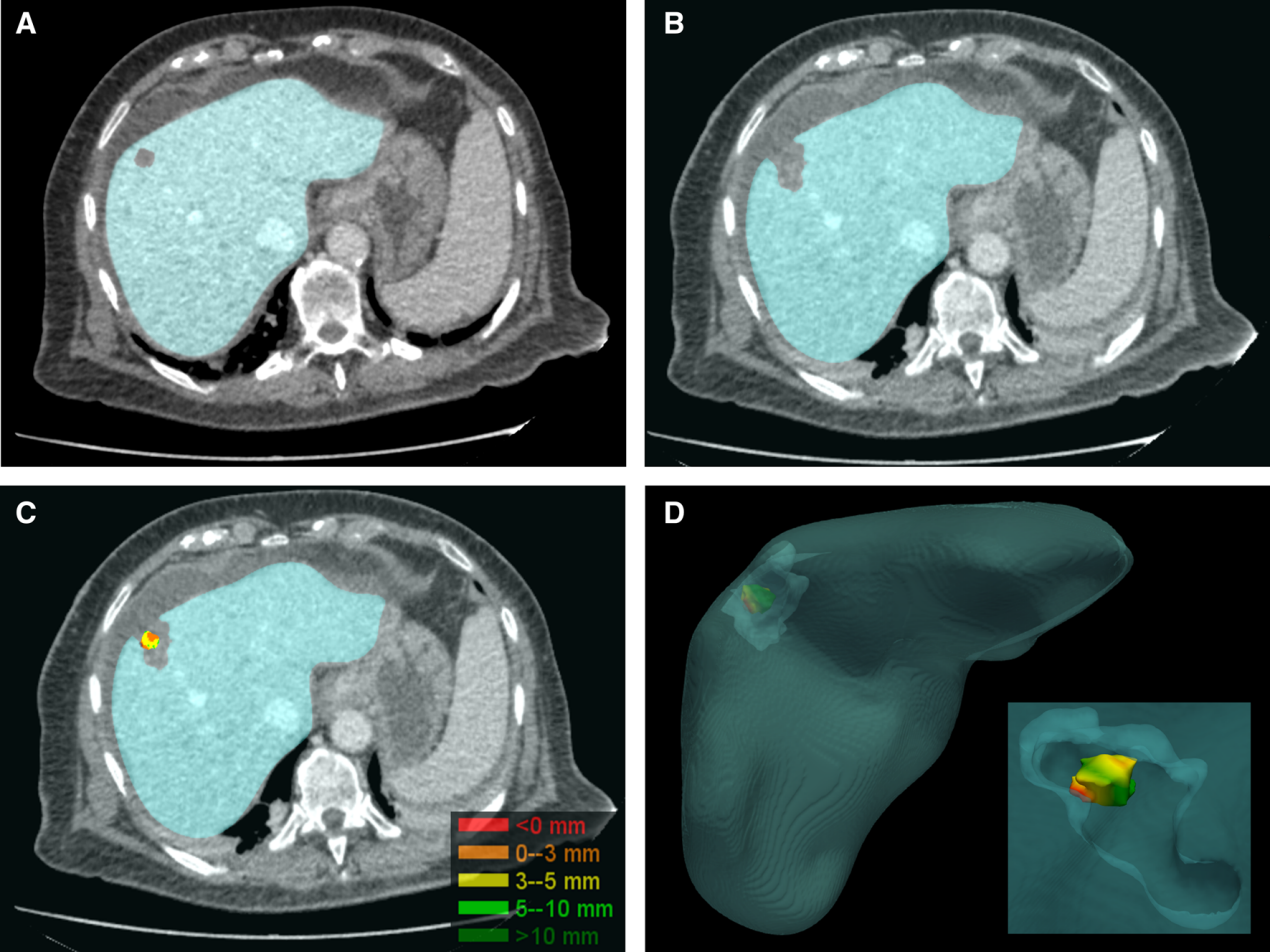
3D Quantitative MAM assessment using deLIVERed in a 78 years old female with a single HCC of 1 cm. A Axial slice showing the liver mask based on delineation of the liver and tumor on the pre-ablation venous phase CT-images. B Axial slice showing the liver mask based on delineation of the liver and ablation zone in the venous phase post-ablation CT-images. C 2D representation of the 3D tumor model with color-coded ablation margins after co-registration. Ablation margins are calculated in 3D, hence showing unexpected tight medial ablation margins which are not visible in 2D. D 3D model of the tumor and ablation zone with color-coded ablation margins after co-registration
